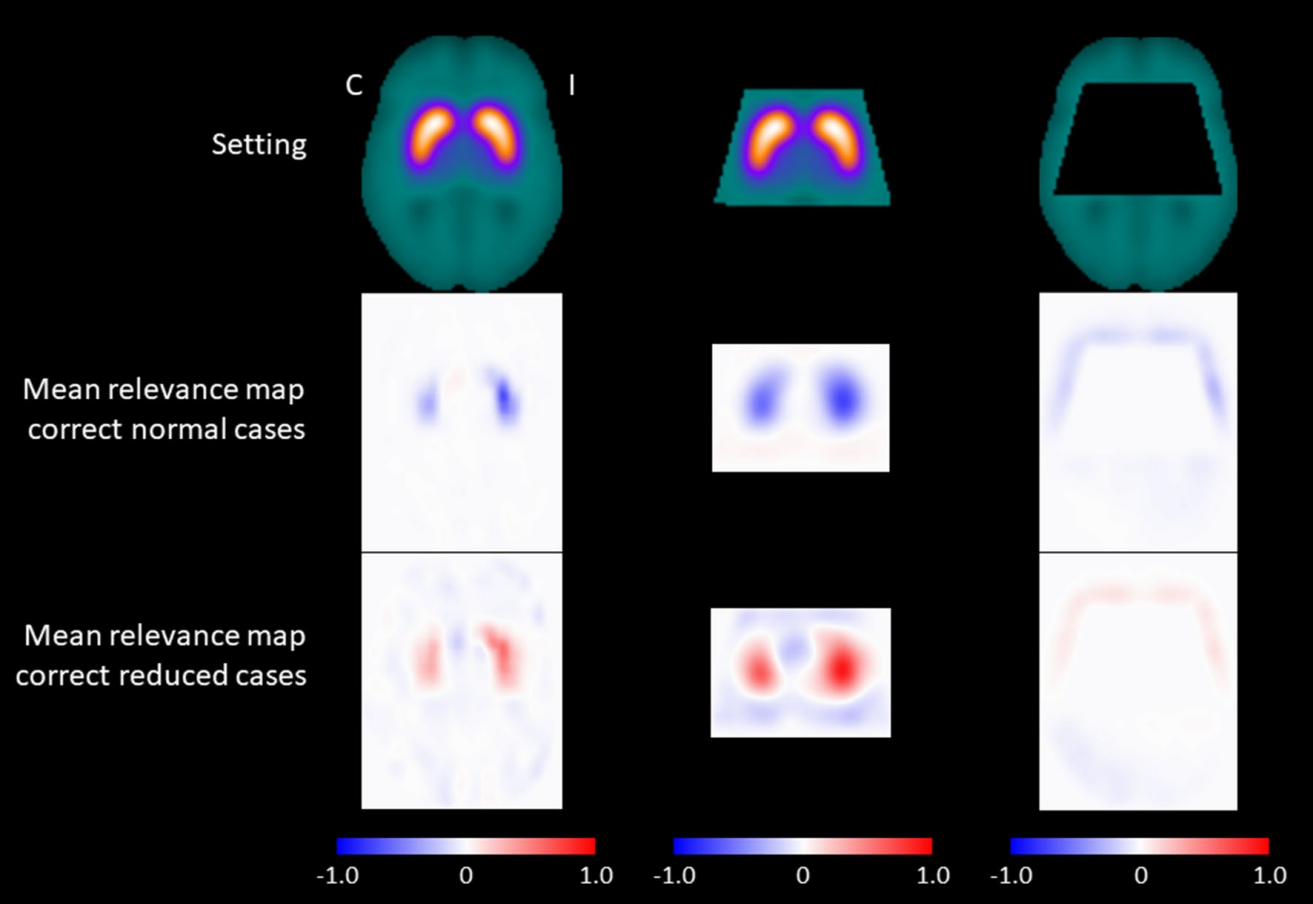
Mean relevance maps. Transversal slice through the striatum of the mean relevance maps of the ^123^I-FP-CIT SPECT images correctly classified as normal (middle row) or correctly classified as reduced (bottom row) by the CNN in the three different settings (“full image”: left column, “striatum only”: middle column, “without striatum”: right column) (*C* contralateral, *I* ipsilateral).
